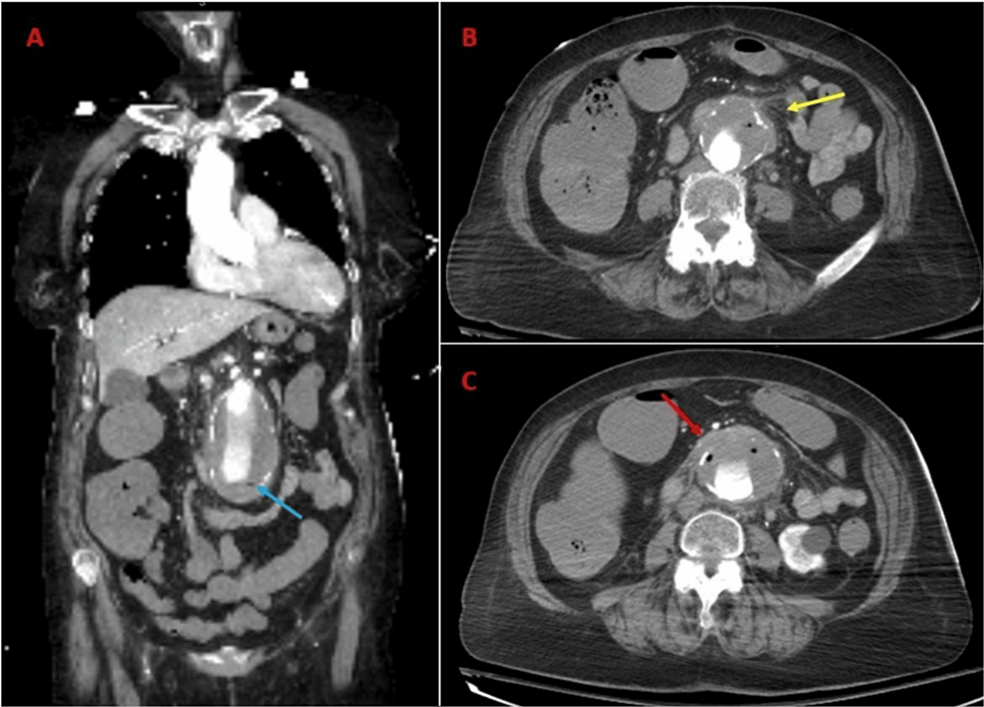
Computed tomography angiography images of Case 2. A and B: Coronal and axial views showing a 5.5-cm abdominal aortic aneurysm with extensive peripheral wall thrombus with a few foci of gas (blue arrow), aneurysm wall thickening, and peri-aortic inflammatory fat stranding (yellow arrow). C: Axial view showing the third portion of the duodenum (red arrow) abutting the aneurysm sac anteriorly.
